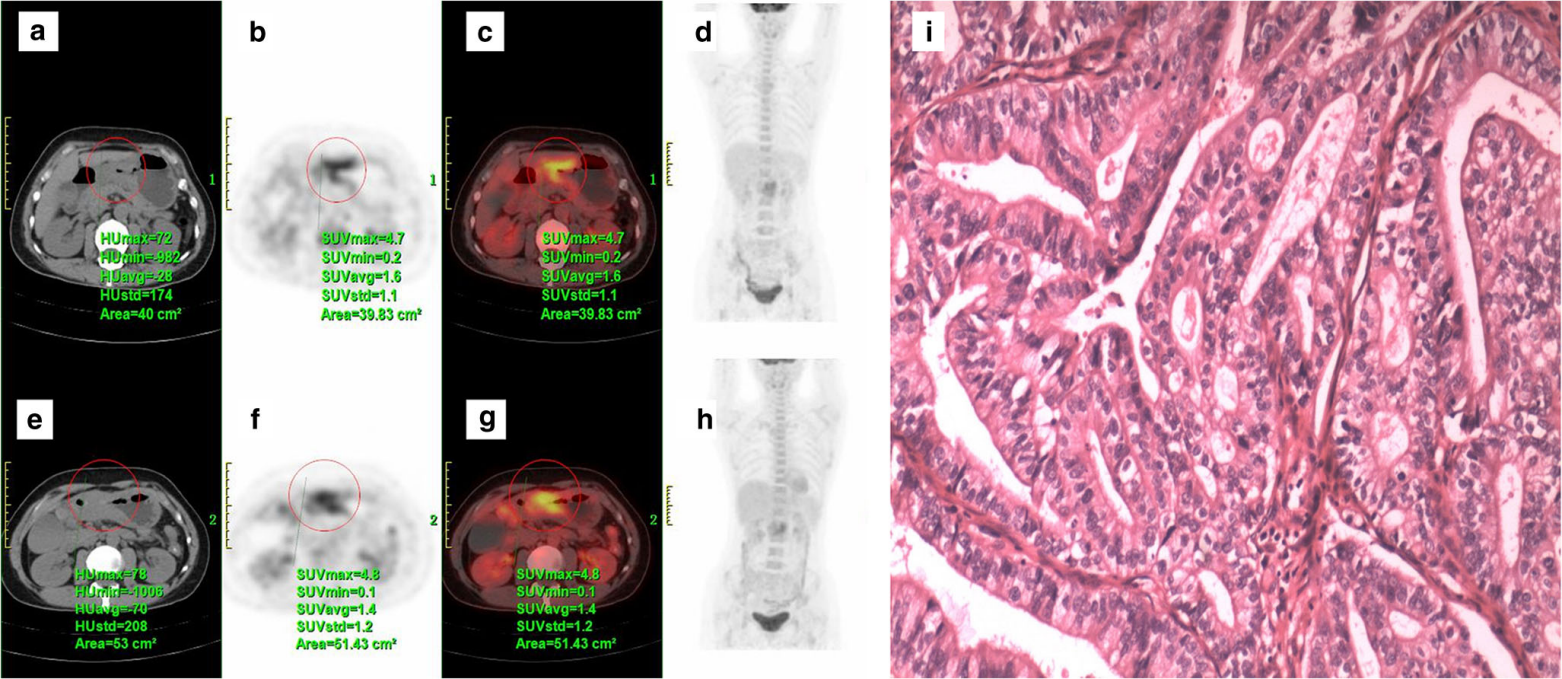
^18^F-FDG PET/CT scan of a 48-year-old woman with gastric cancer (a–d) demonstrated by an intense uptake of ^18^F-FDG before treatment (SUV_max_ = 4.7) (c). After one cycle of chemotherapy (capecitabine + paclitaxel) (e–h), no significant changes in ^18^F-FDG uptake (SUV_max_ = 4.8) were observed (g). Final pathology (i) ypT4aN1 (tumour regression grade 3)
